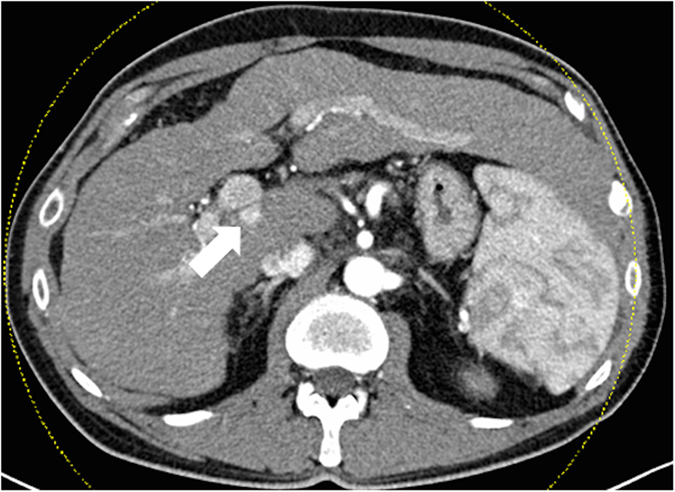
52-year-old patient with hepatocellular carcinoma. Pre-interventional computed tomography for intervention planning: arterial hypervascularized mass on the transition to liver segment I posterior to the main stem of the portal vein.
